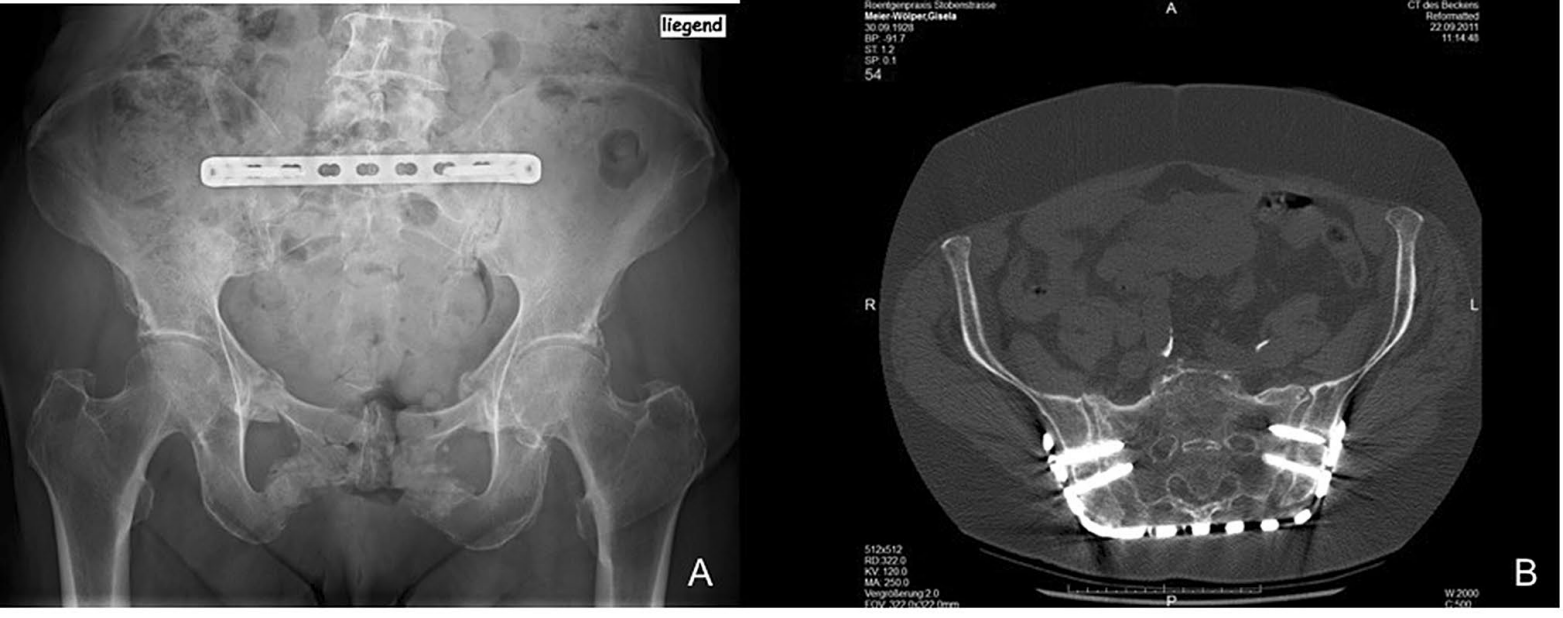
Follow-up X-ray and CT scan
